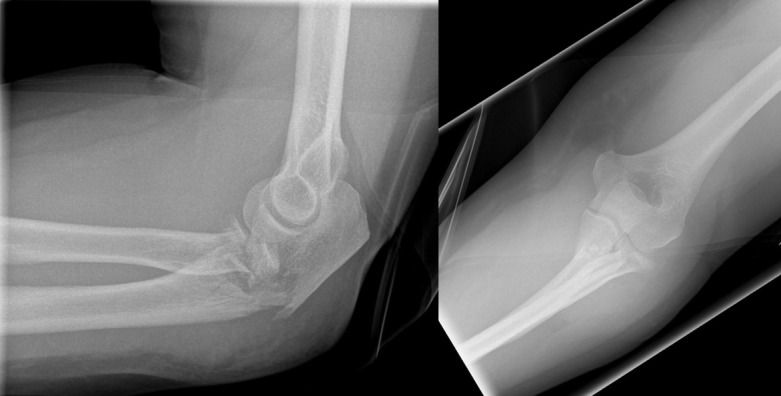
Male patient (43 years) with a posterior Monteggia-like lesion (Bado type II, Jupiter type IIB), Mason type III fracture of the radial head, and Broberg and Morrey type III coronoid fracture. Radiographs on the day of the injury.
